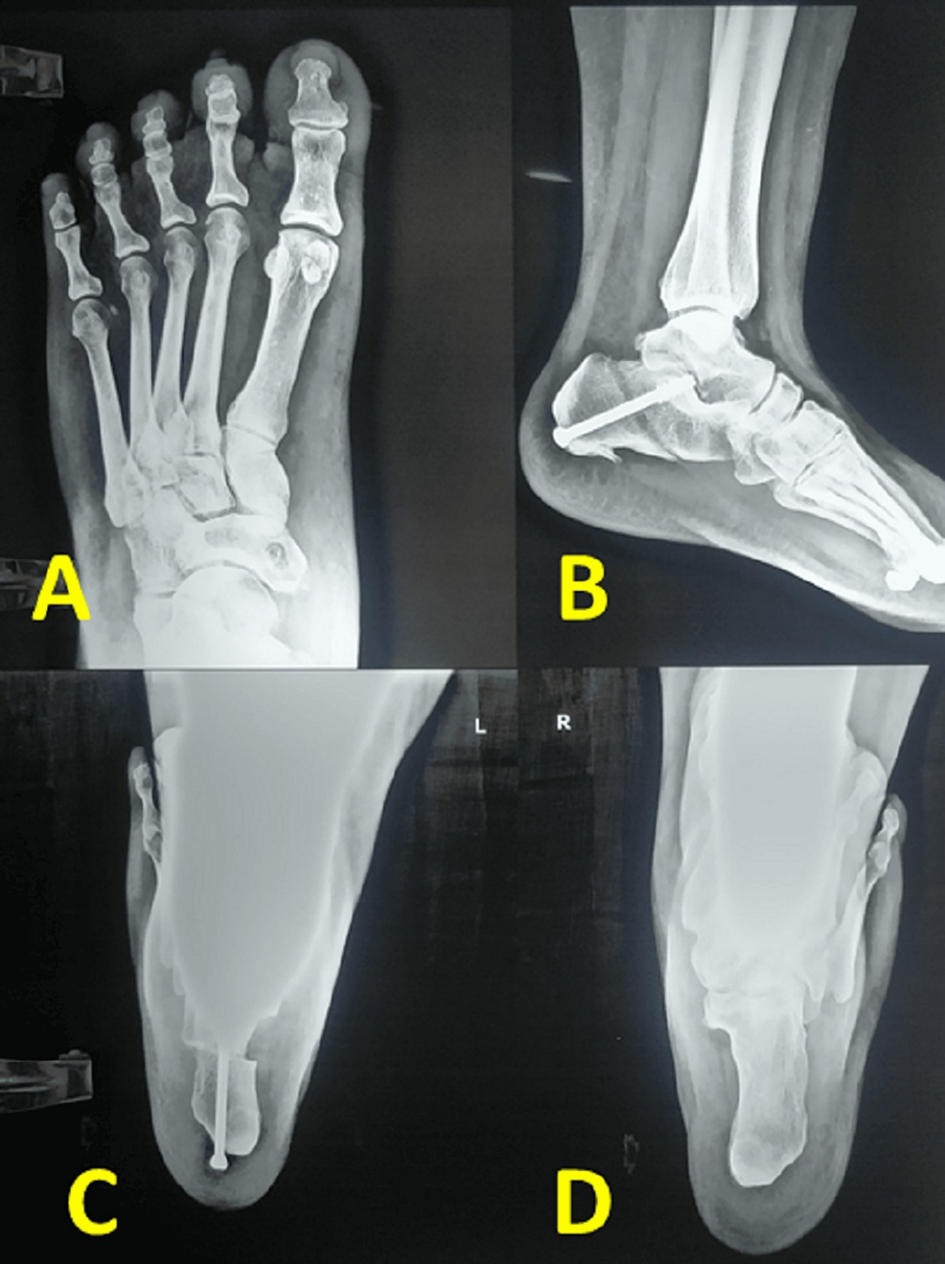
Postoperative X-ray of a patient who had undergone the quadrangular construct and medial displacement calcaneum osteotomy Figure A shows a hole around the navicular tuberosity, indicating the navicular tunnel of a quadrangular construct. Figures B and C show a medial displacement calcaneum osteotomy fixed with a single screw. Figure D shows a comparative Saltzman view of the normal side [4].
